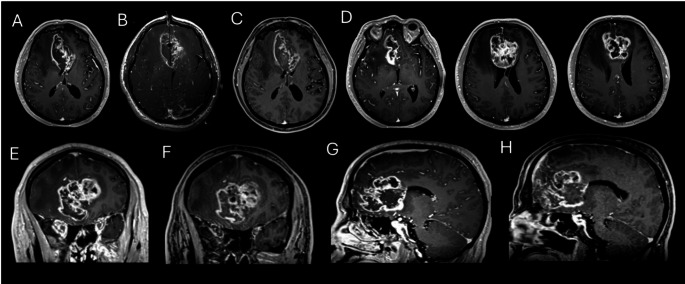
An example of an unfavorable shape for successful large-volume ablation in LITT. High pre-operative volume (69.4cm^3^), with a low subsequent ablation volume (7.50cm^3^) and a low EOA (11%) with a high RTB of 61.87cm^3^. The multiple loculations, closeness to heatsinks such as ventricles, dural folds and vasculature and the lack of an even, cylindrical structure all contribute to the overall EOA. Nevertheless, this patient achieved an OS of 19.3 months (censored) and a PFS of 11.05 months, suggesting that it is not as clear cut as smaller EOA causing worse survival in this aggressive subtype of tumors. A – Axial pre-operative T1 with contrast MR image. B – Laser catheter approach intra-operatively. C – Post-ablation scan showing ablation cavity enveloped by residual tumor. D – Further pre-operative axial T1 plus contrast image showing the large extent of the lesion. E – Pre- and F – Post-operative coronal T1 with contrast MR image. G – Pre- and H – Post-operative sagittal T1 with contrast MR image. All post-operative images taken on POD1
